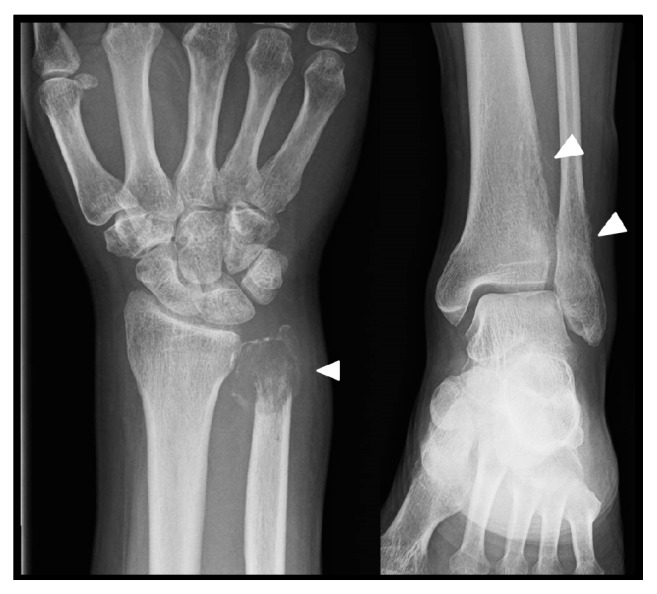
Plain radiographs of right distal ulna and the left lower leg show irregular osteolysis.
